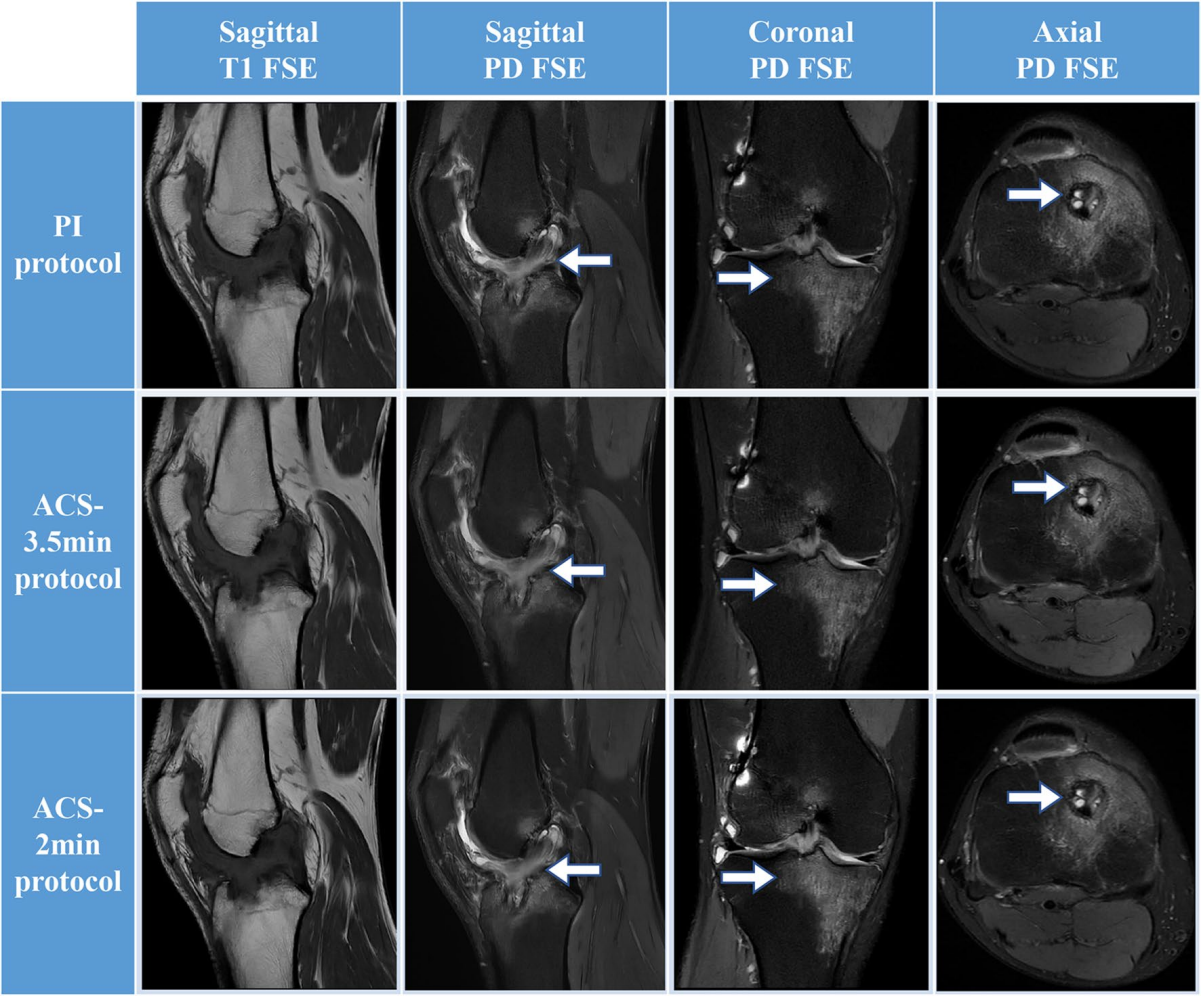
MRI images of a 23-year-old male after anterior cruciate ligament (ACL) reconstruction. From left to right, sagittal T1-weighted images, proton density–weighted fat-suppressed (PDFS) sagittal, coronal, and axial images of fast spin-echo (FSE) imaging, respectively. T1-weighted images show bone condition; sagittal PDFS shows ACL continuity (arrows); coronal PDFS shows bone marrow edema (arrows); transverse PDFS shows bone tract and bone marrow edema of the tibia (arrows)
